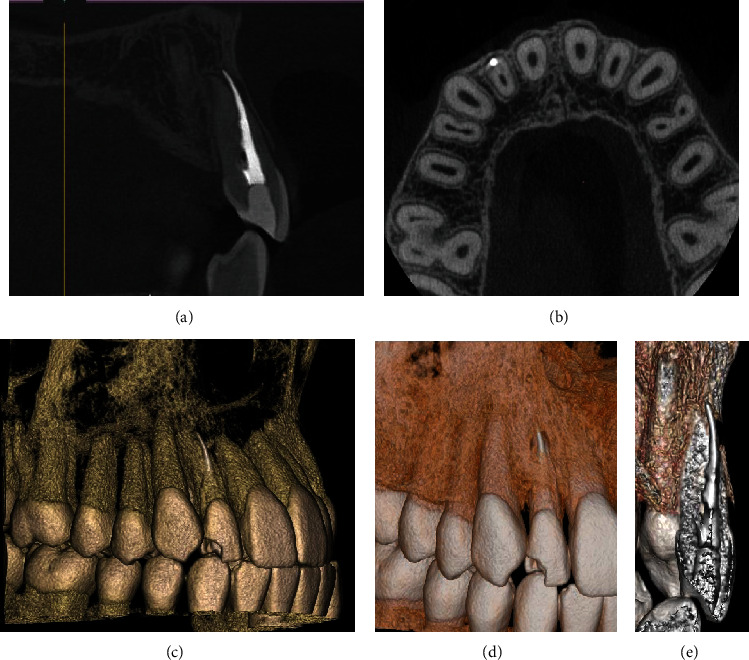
CBCT scans (a–e) and 3D reconstructions show sealing material out of the channel, extruded by root perforation in the middle third of the root canal.
